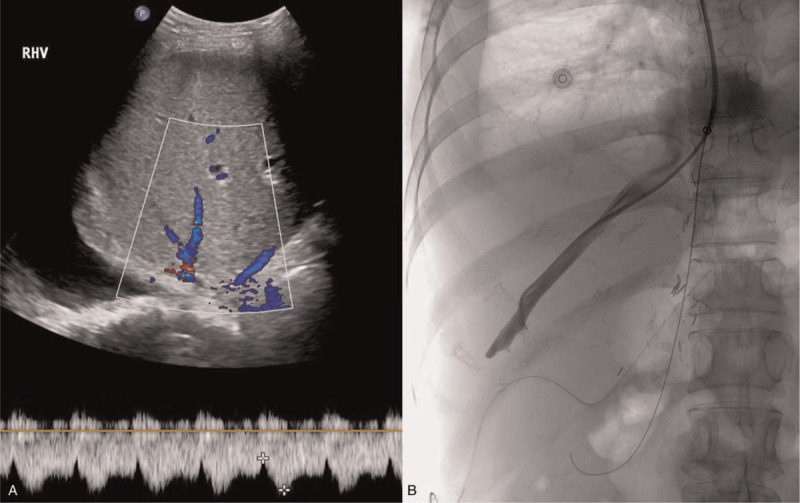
(A) Doppler ultrasound image showing normal hepatic hemodynamics without obstruction of hepatic veins on postoperative day 14. (B) Transjugular hepatic venography on the same day also shows no obstruction or stenosis of the right hepatic vein.
